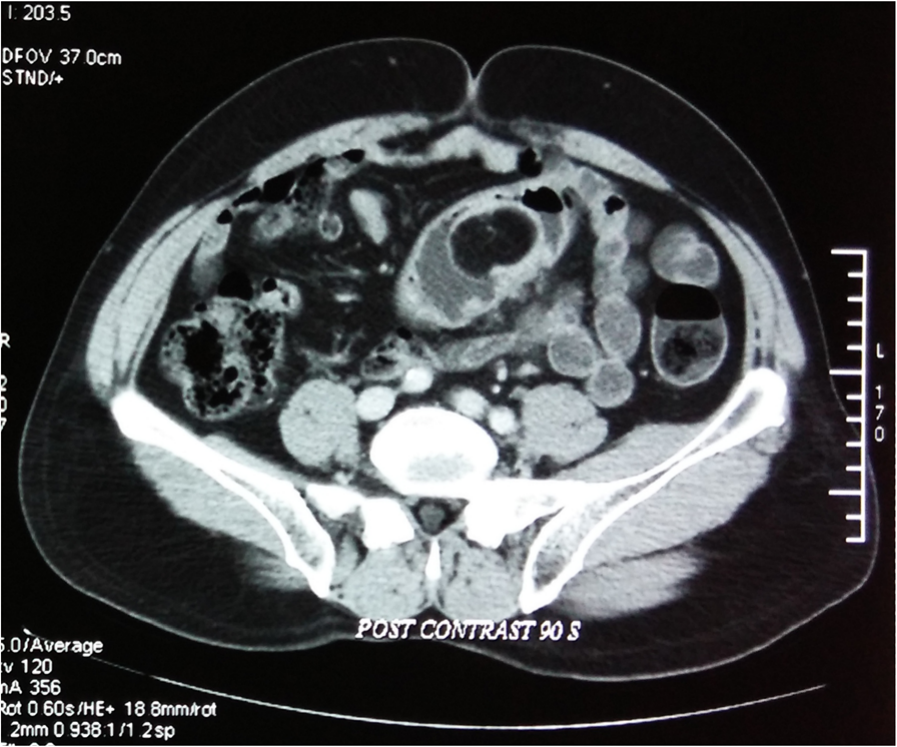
Contrast-enhanced computed tomography scan showing a hypodense lesion in the sigmoid colon
